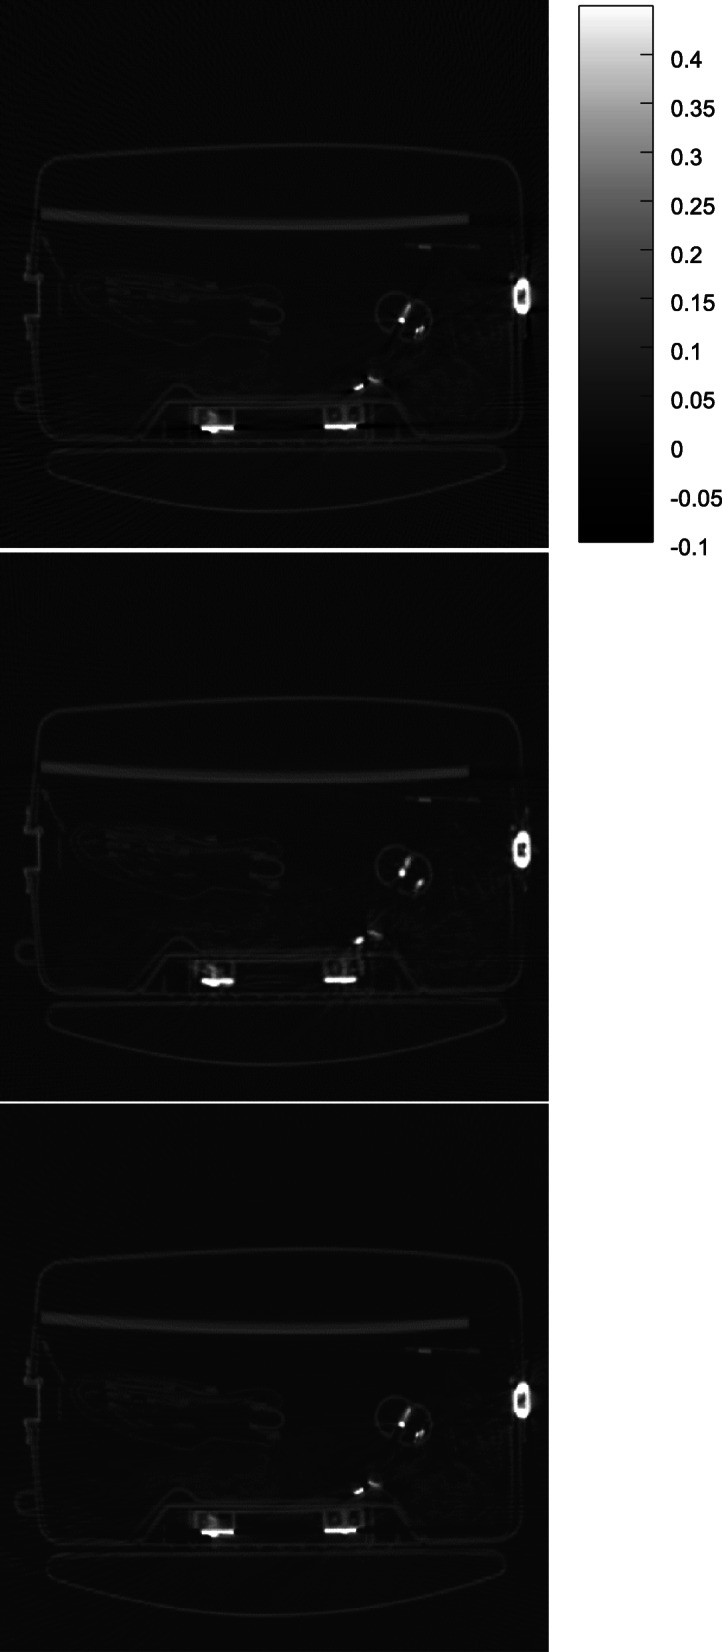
CT image reconstruction of airport bag #3. Top: the raw FBP reconstruction; Middle: the reconstruction using the proposed algorithm; Bottom: the raw FBP reconstruction with all negative pixel values replaced by zeros
